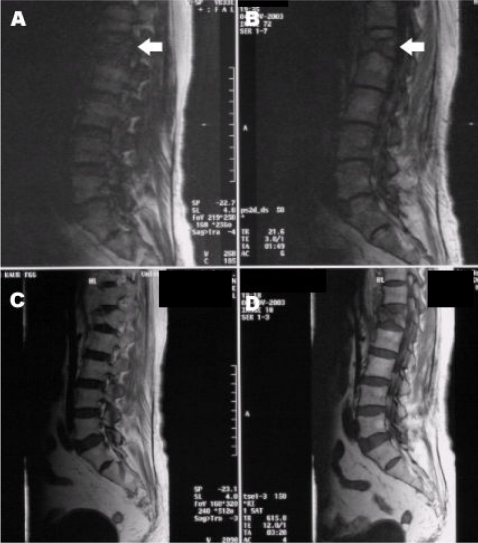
Mid sagittal MR images of the spine with an osteoporotic fracture of L1 vertebral body. Mid sagittal SSFP DWI MRI at a TE of 5ms (A) and 3ms (B) show low signal intensity (arrow) in the collapsed L1 vertebral body compared to the normal bone marrow in adjacent vertebral bodies. There is no contrast enhancement of the L1 vertebra (C) after gadolinium administration.T1 W MR image (D) shows low signal in the L1 vertebra.
